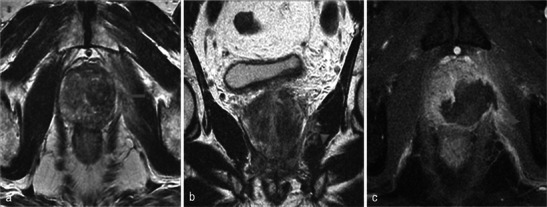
Internal obturator muscle. Left photodynamic therapy in a 66-year-old patient with Gleason 6 adenocarcinoma (pre-treatment/day-7 post-treatment/6-month post-treatment). Axial (a) and coronal (b) T2-weighted MR images show high signals of the left internal obturator muscle (*arrowhead*) and the puborectalis muscle (*arrow*). Extraprostatic necrosis extending to muscles is well defined on the T1 FS contrast-enhanced image (c, flash)
